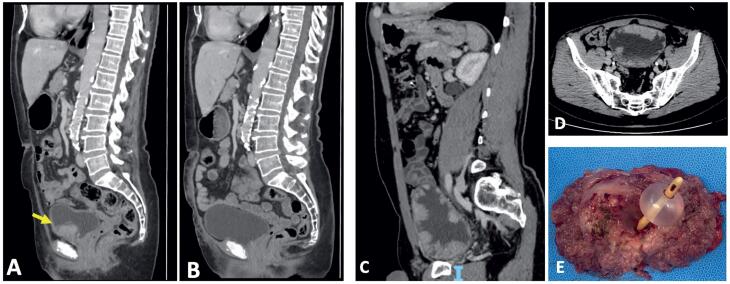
Computed Tomography (CT) scan of a patient who underwent a partial cystectomy for an anterior bladder neck LG-NMIBC (yellow arrow) could not be adequately resected endoscopically. A) preoperative image. B) After 22 months of follow-up. Radical cystectomy was performed for an extensive LG Ta NMIBC. C) and D) CT scans demonstrating extensive LG-Ta lesion. E) surgical aspect of the bladder after radical cystectomy.
